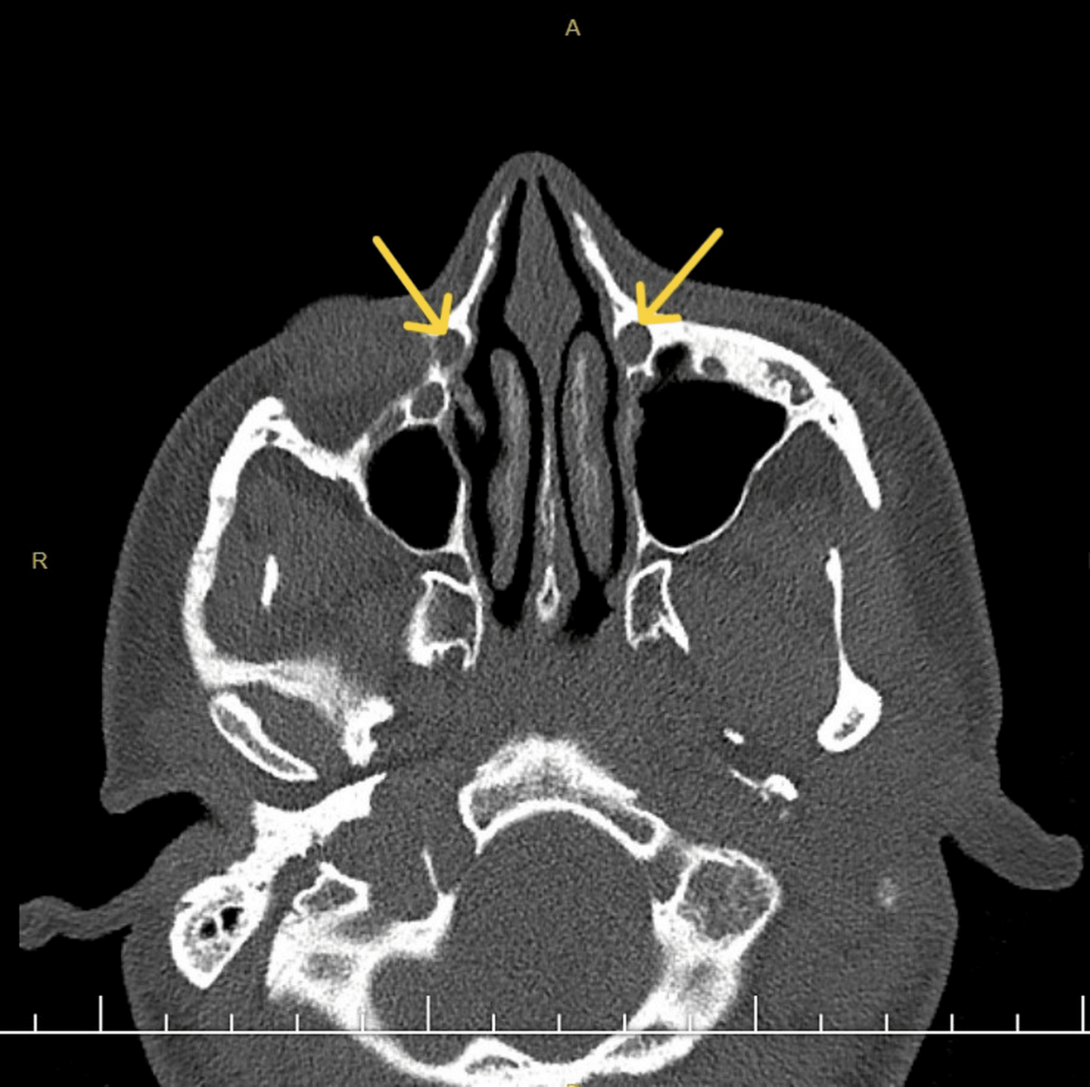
In the horizontal paranasal CT, left inferior turbinate hypertrophy are observed. Yellow arrow points to the nasolacrimal duct.
